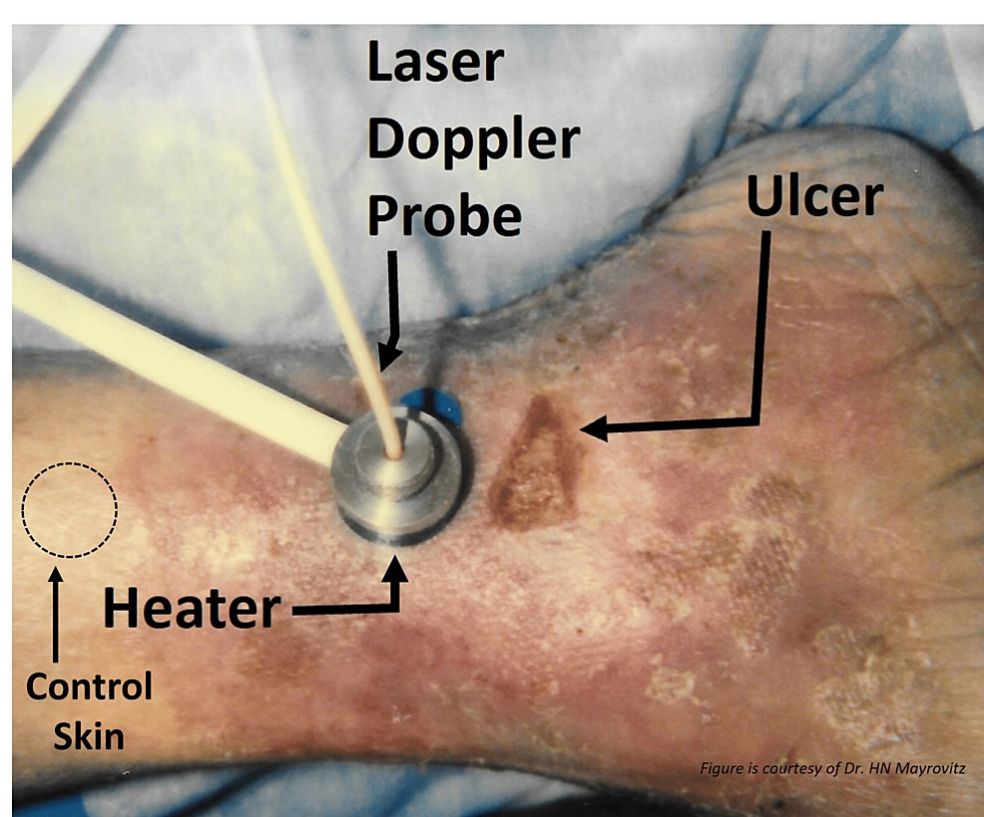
Periulcer skin blood perfusion measurement A laser Doppler probe is fitted through a concentric hole in the heater that is in contact with the skin. Localized heating produces an increase in microvascular perfusion in the healthy skin but with a different pattern in the peri-wound skin as shown in Figure 4. This figure is provided as a courtesy of Dr. HN Mayrovitz.
